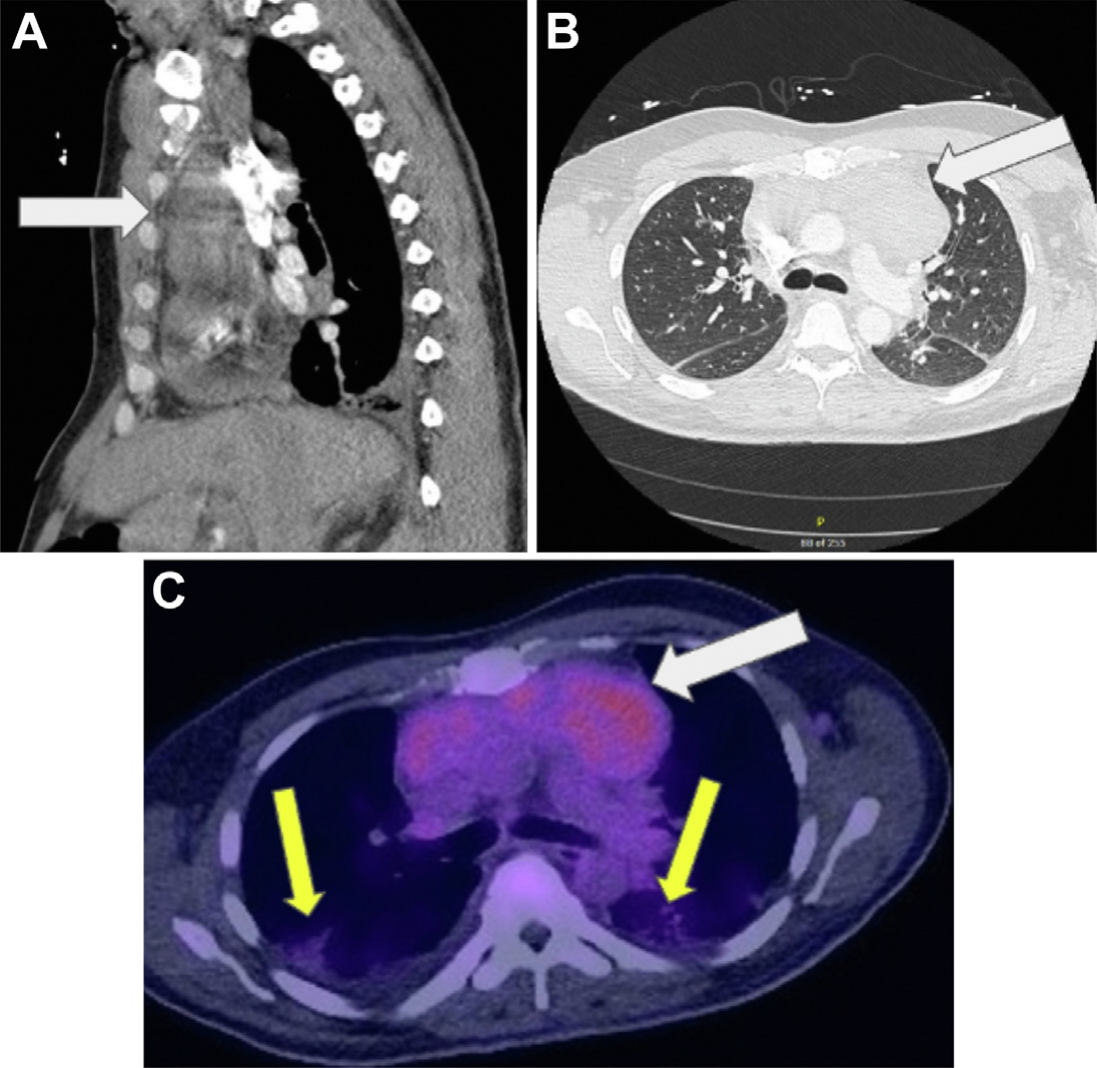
(A) Sagittal chest computed tomography (CT). (B) Axial chest CT. (C) Axial chest CT/positron emission tomography scan. The white arrows point to the anterior mediastinal mass, and the yellow arrows point to pleural effusion.
